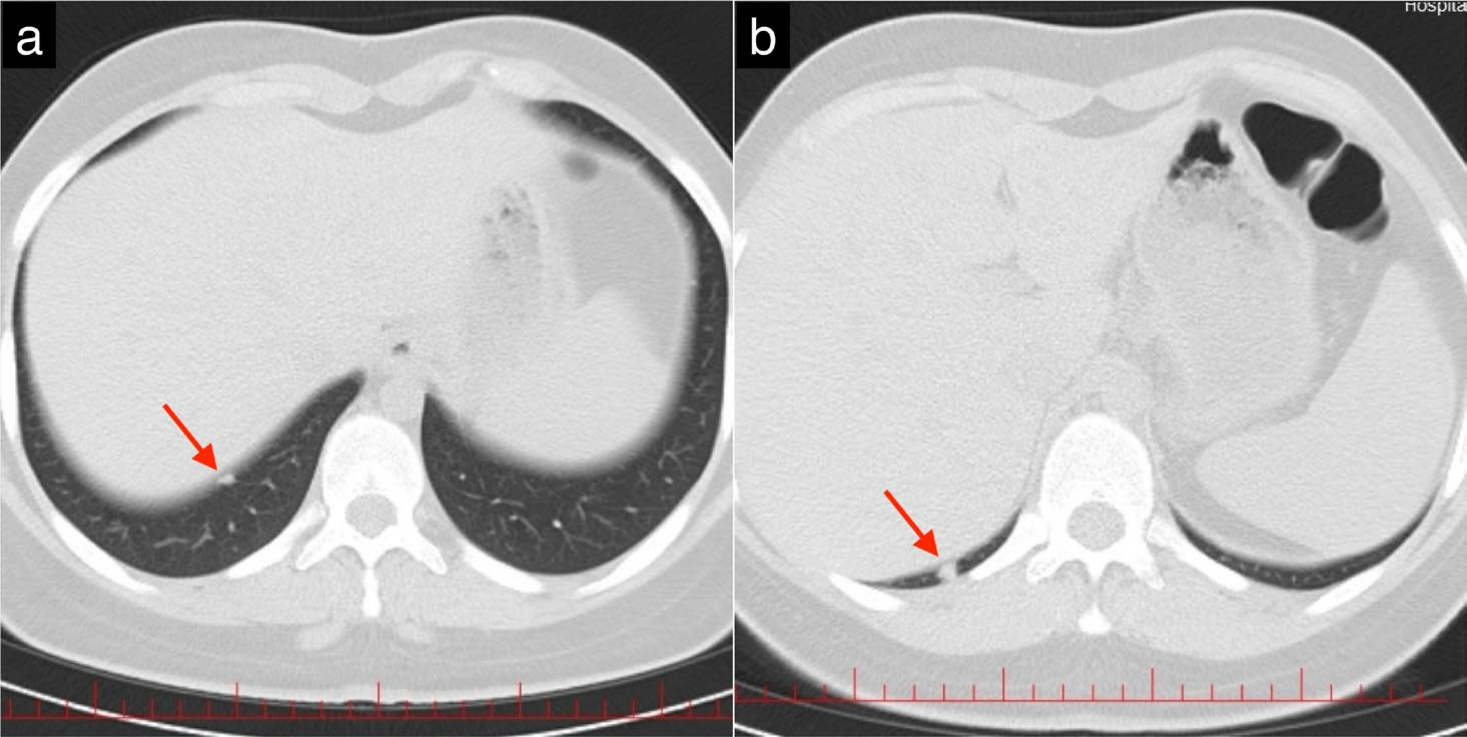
HRCT of lungs. (a and b) Well‐circumscribed parenchymal nodules (red arrows).
